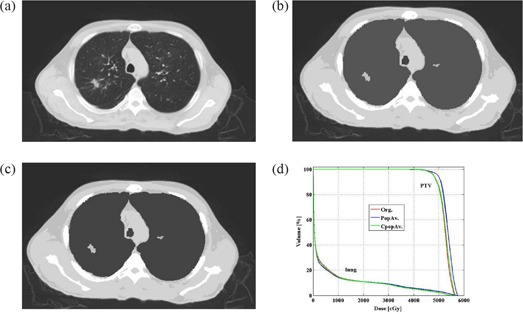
An example of a patient with emphysema. Fig.3(a) original treatment planning CT image (lung window); (b) treatment planning CT image of the same slice as with the 5 bulk densities applied; (c) treatment planning CT image of the same slice as Figs. 3(a) and (b) where the individual average density is applied to the lung; (d) DVH comparing the PTV and lung of the 3 plans.
